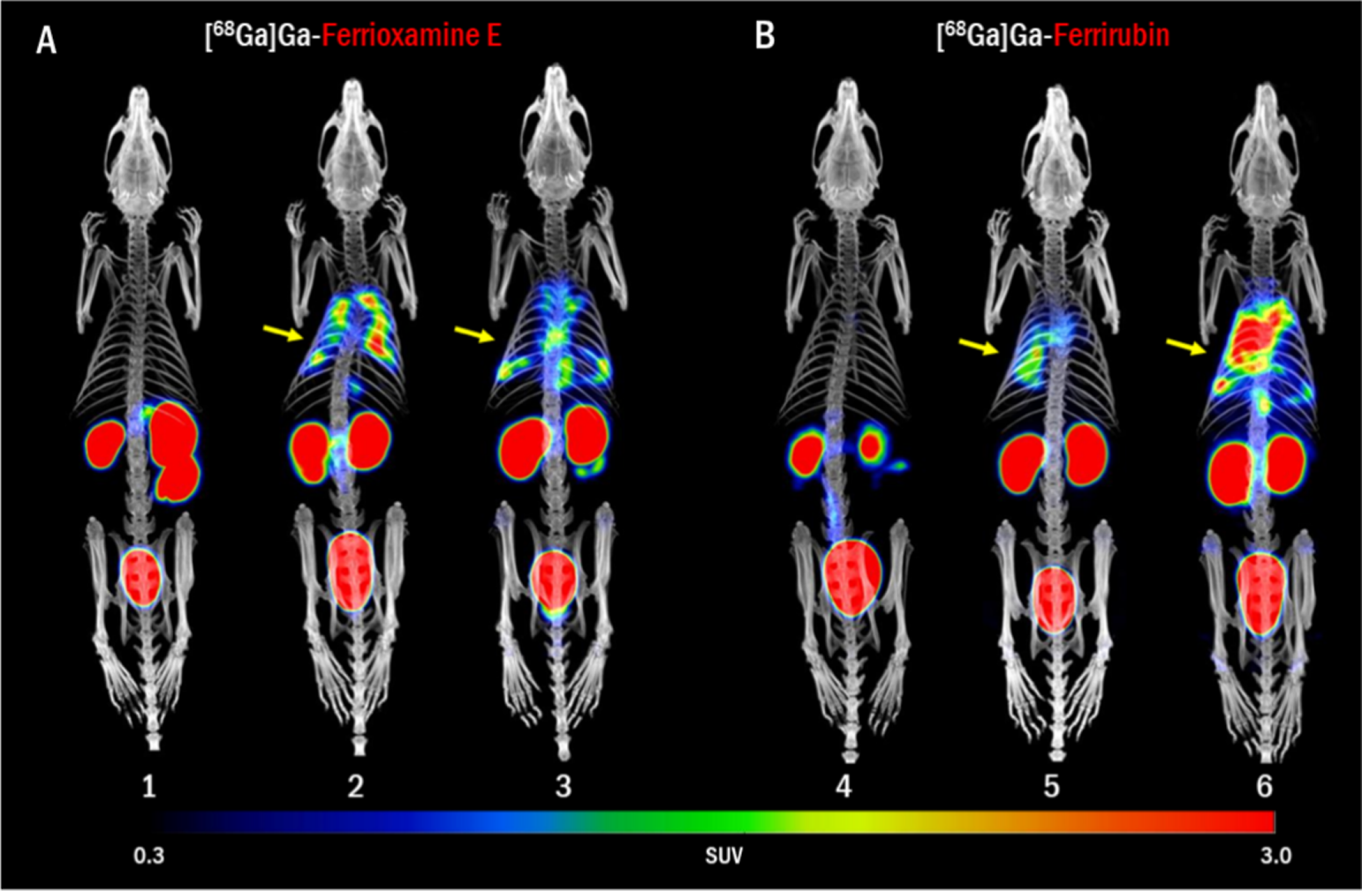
PET/CT in vivo imaging of (A) [^68^Ga]Ga-FOX E and (B)
